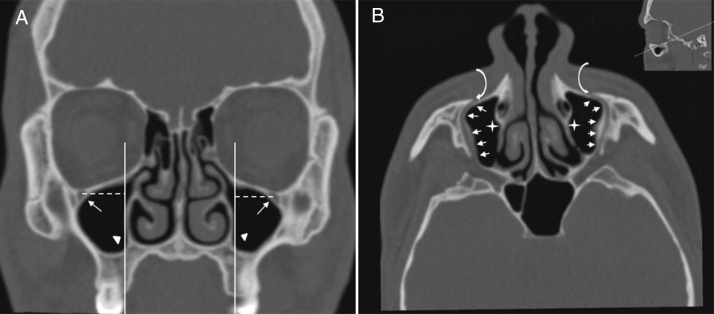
(A) Coronal paranasal sinus CT image showing bilateral infraorbital canal Type 4 which is located at the outer limit of the zygomatic recess and the horizontal distance (white dashed line) from the center of the IOF (arrows) to the plane passing through the priform aperture (arrowheads). (B) An oblique/axial image showing bilateral lateroantral canals (arrows) which are identified coursing laterally to the maxillary sinuses (asterisks) and opened by infraorbital foramina (curved arrows).
